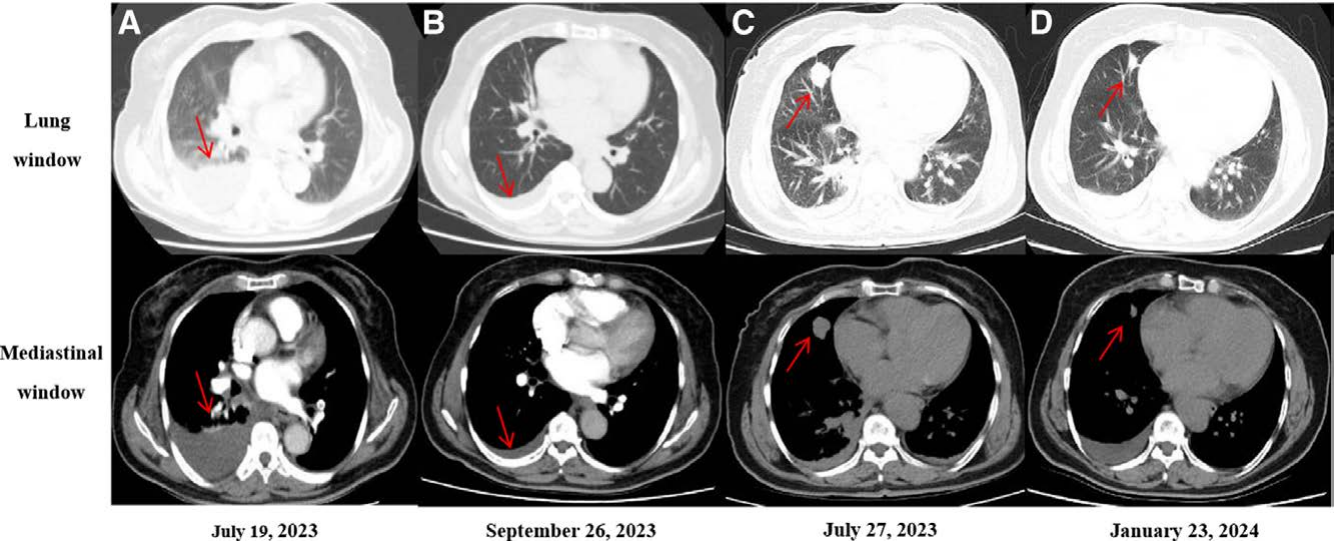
A pretreatment, July 19, 2023: (A) CTA of the pulmonary artery demonstrated the presence of emboli in the left pulmonary artery, alongside partial consolidation and atelectasis in the middle and lower lobes of the right lung, as well as a right-sided pleural effusion. (B) Post-anticoagulant therapy, September 26, 2023: subsequent to the initiation of anticoagulant therapy, an evaluation of the pulmonary artery CTA revealed residual emboli in the left pulmonary artery, persistent partial consolidation in the lower lobe of the right lung, with no significant changes noted, and ongoing pleural effusion on the right side. (C) Pre-targeted therapy, July 27, 2023: prior to the commencement of targeted therapy, a chest CT scan revealed a space-occupying lesion in the right lung, measuring approximately 23 mm by 18 mm. (D) Six months post-targeted therapy, January 23, 2024: after 6 months of targeted therapy, a follow-up chest CT scan showed a reduction in the size of the pulmonary lesion to approximately 15 mm by 8 mm. CT = computed tomography, CTA = computed tomography angiography.
